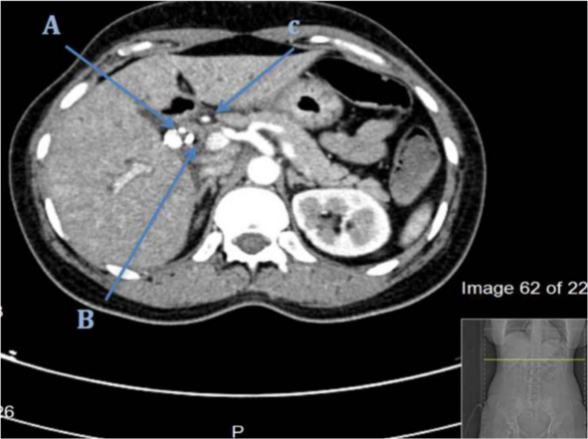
CT study, an axial cut: the arrows points at, A: right hepatic artery psuedoaneurysm B: Stent C: common hepatic artery
